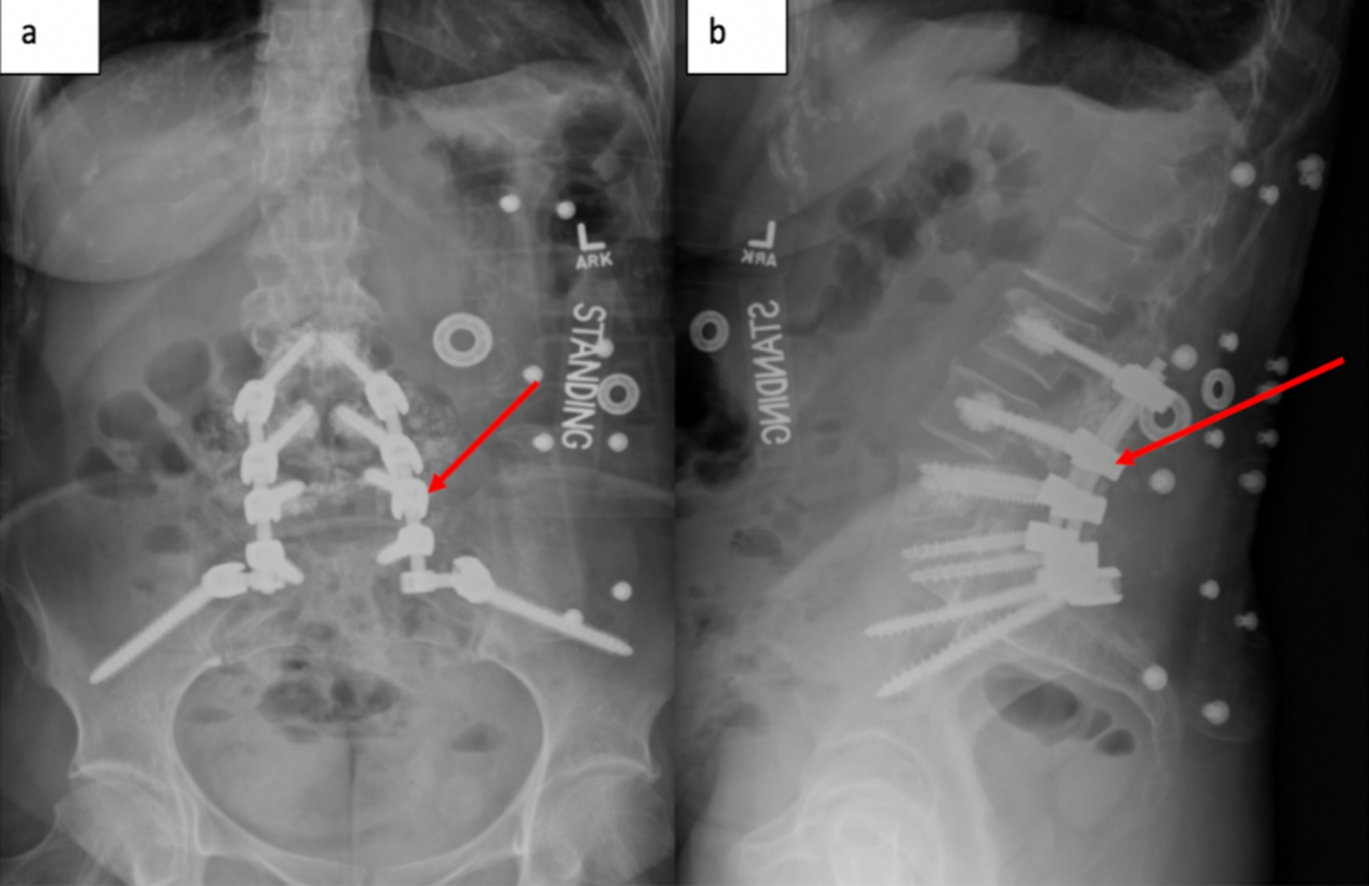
Plane films of the spine Postoperative AP (a) and sagittal (b) X-rays demonstrate the L3-S1/pelvis instrumentation. Standing - Patient is in the standing position.
